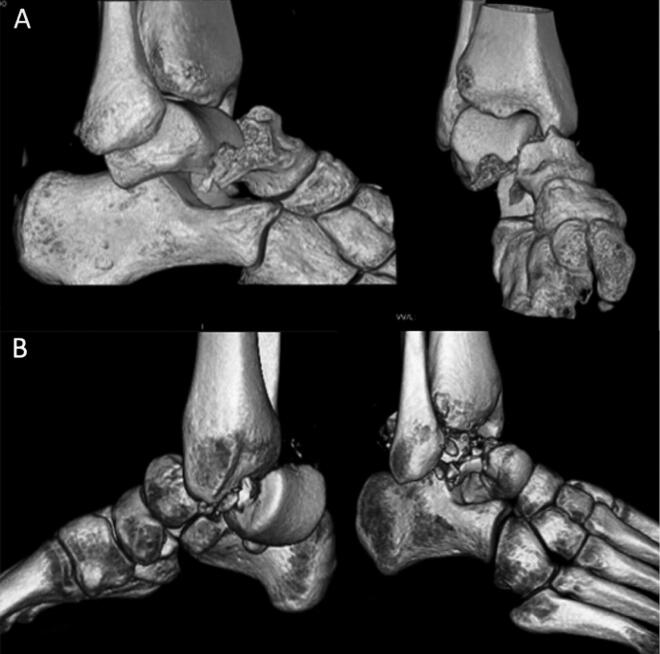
3-D CT reconstruction of fracture types represented in the study population: Hawkins type 2b (A) and type 3 (B).
